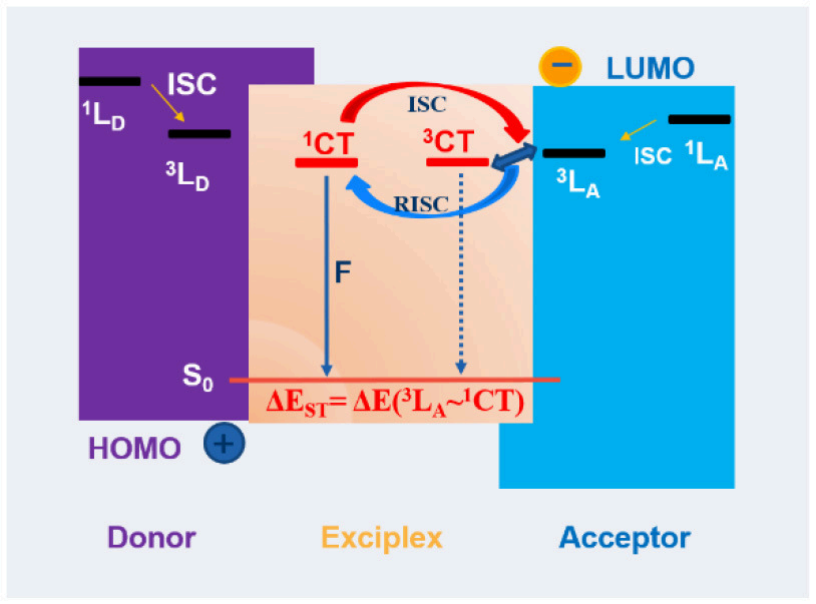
Design and prediction of the highly efficient exciplex emitters is desirable to optimize the ^1^CT, ^3^CT of the exciplex and ^3^LE of the donor or accepter. Here, we studied the newly molecule TX-TerPy as the acceptor blended with TAPC, TCTA and m-MTDATA. The energy gap between the ^1^CT and ^3^LE in TCTA: 15 wt% TX-TerPy blends film is as small as 0.19 eV. So the Φ_PL_ is the highest one and nearly 100% triplet exciton can be obtained.
